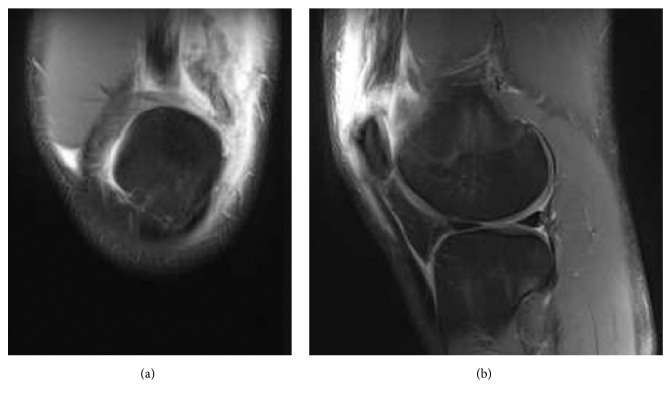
Coronal (a) and sagittal (b) representative magnetic resonance imaging (MRI) images denoting a quadriceps rupture in an otherwise healthy 17-year-old competitive athlete.
